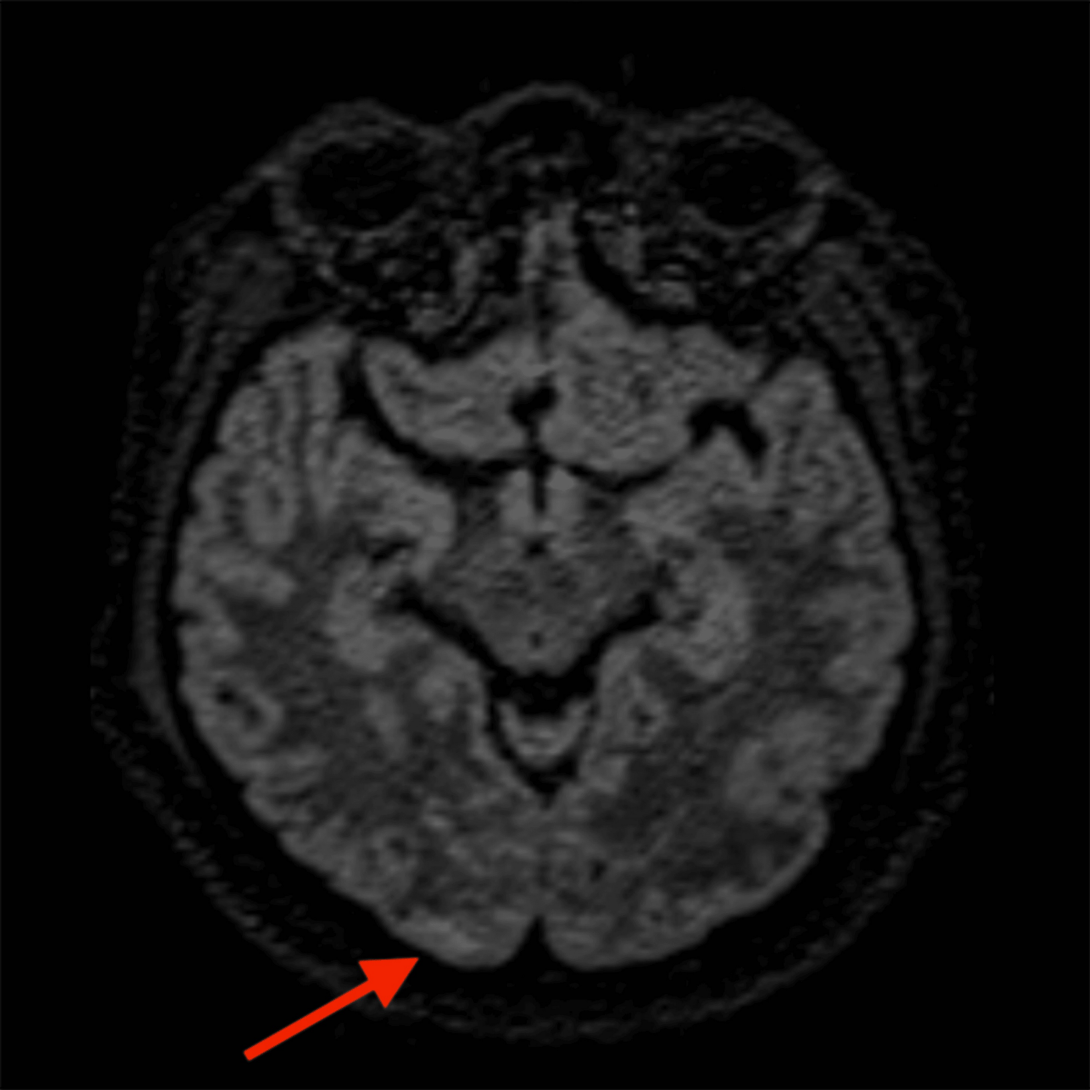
Axial FLAIR image of Patient 1 (AS) demonstrating hyperintensity (arrow) in the occipital region The red arrow points to the occipital region with patchy FLAIR hyperintensity, consistent with PRES FLAIR: fluid-attenuated inversion recovery; PRES: posterior reversible encephalopathy syndrome
